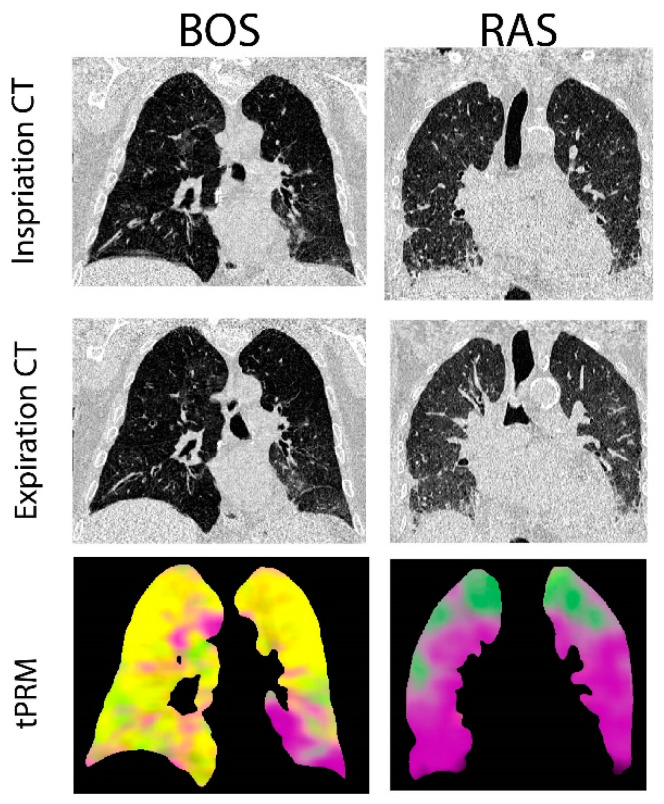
Representative cases diagnosed with BOS and RAS. Presented are the coronal view of CT scans acquired at inspiration and expiration with corresponding topological parametric response mapping (tPRM)-derived volume density maps for normal parenchyma (green), functional small airways disease (fSAD; yellow) and parenchymal disease (PD; magenta).
